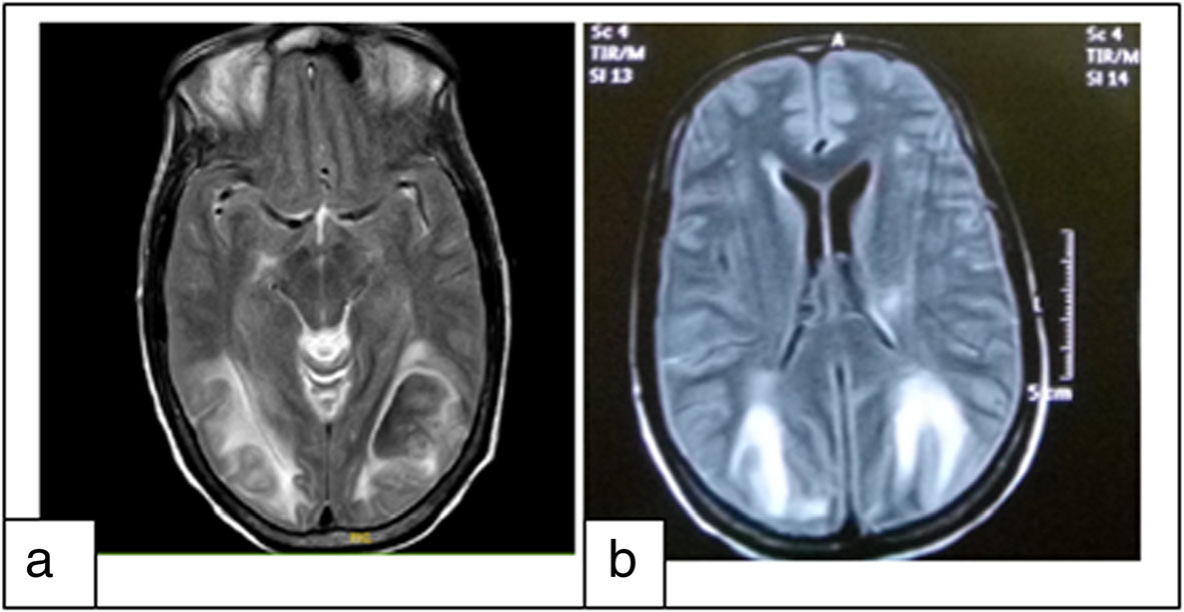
MRI findings (T2 sequence (a), FLAIR (b)) showing the diffuse confluent white matter hyperintensities in bilateral parieto- occipital region
